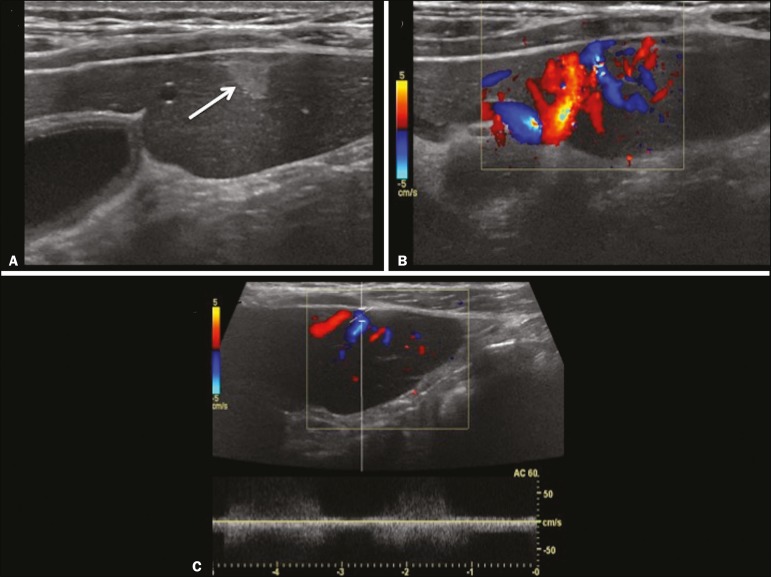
Congenital hepatic arteriovenous malformation in a 4-year-old girl with hereditary hemorrhagic telangiectasia who presented with complex hepatic vascular malformation. A: B-mode ultrasound shows subcapsular hyperechoic focal lesion (telangiectasia). B: Doppler ultrasound showing tortuous large vessels towards the lesion. C: Spectral ultrasound analysis showing turbulent flow at high velocity. Signs of portal hypertension, such as reversal of the portal venous flow and collateral circulation, were present at diagnosis (images not included).
